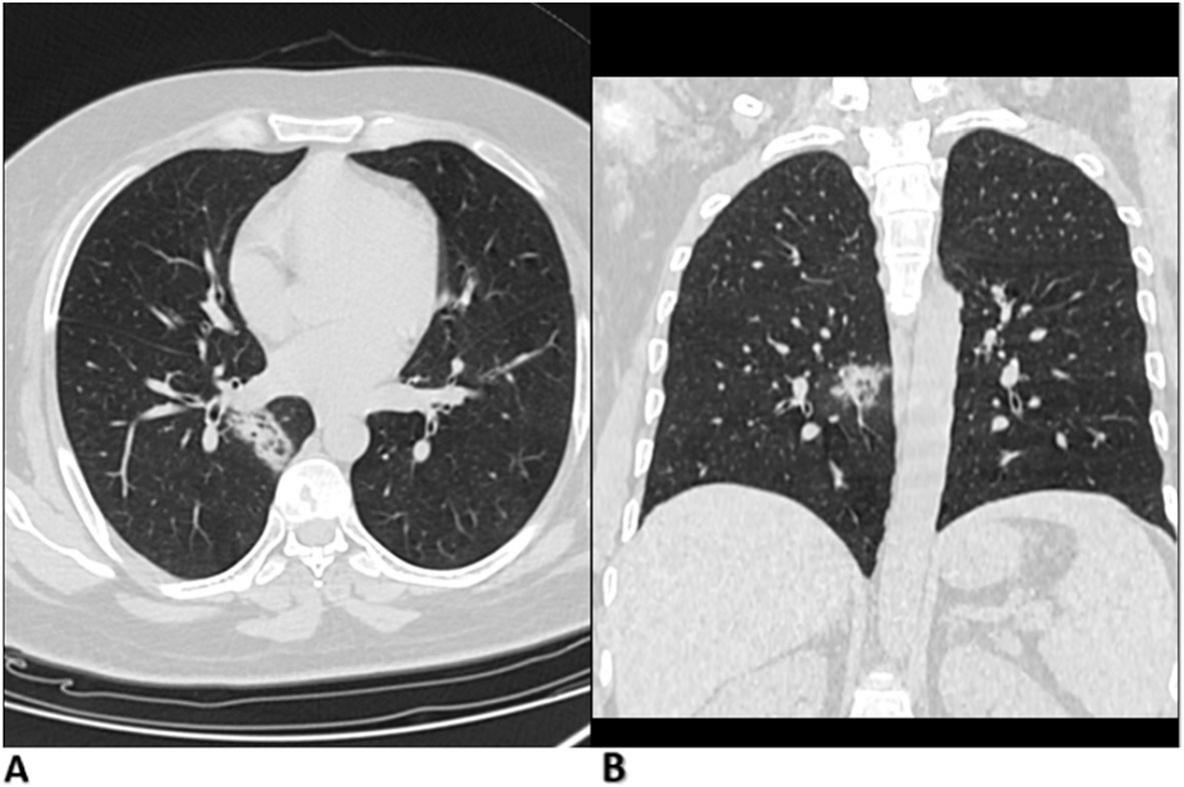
A 36-year-old man with CT findings of single ground-glass opacity. Observers interpreted 3 and 4 CO-RADS scores and agreed on COVID-RADS score 2A. The preference was in favor of COVID-RADS to CO-RADS (3:0). RT-PCR result was positive
